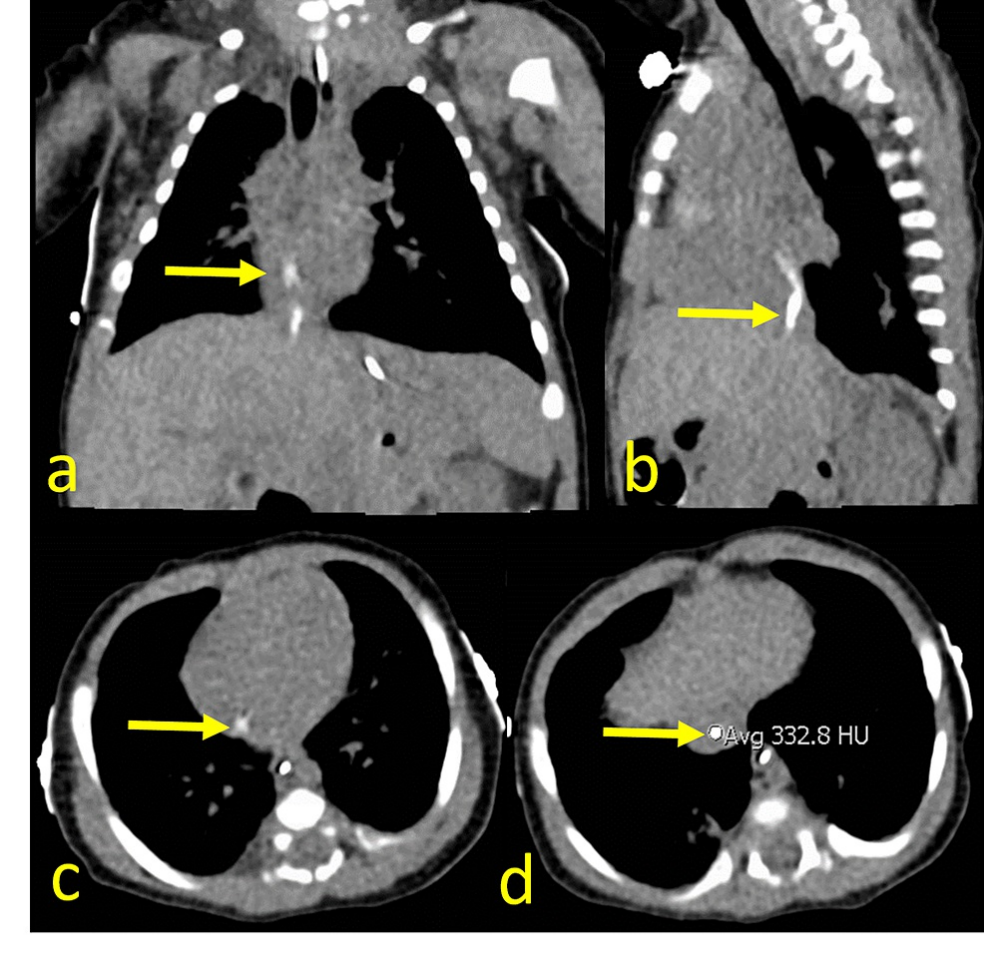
Coronal (a), sagittal (b), and axial (c, d) CT images on day 48 demonstrate a non-occlusive curvilinear hyperdensity extending from the intrahepatic inferior vena cava to the right atrium (arrows). Incidental note of enteric tube in the esophagus and stomach
